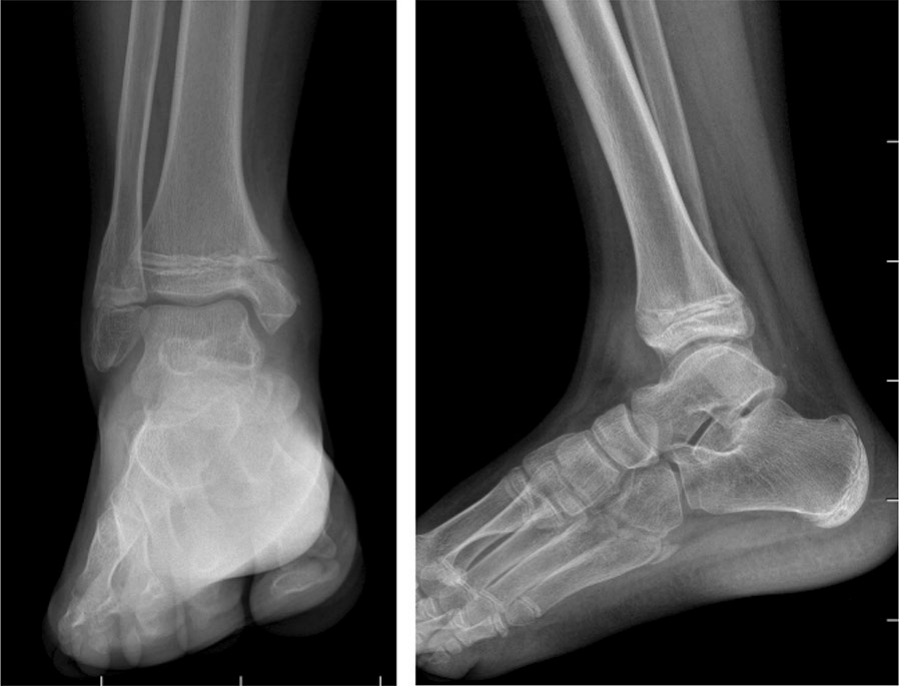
X-rays after implant removal 6 months after operation
